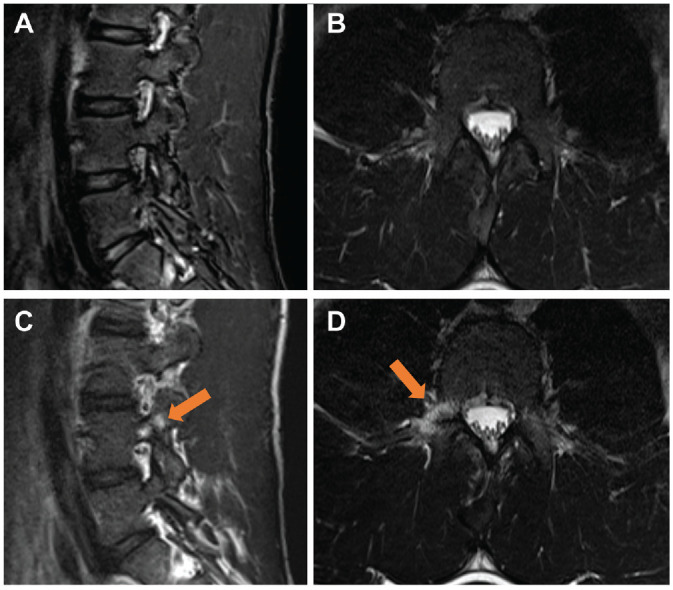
Sagittal Dixon and transverse T2-weighted magnetic resonance imaging scans of the lumbar spine of a 19-year-old competitive alpine skier (A and B, respectively) at baseline and (C and D, respectively) at the 48-month follow-up. Note the bone marrow edema in the right facet joint of the fourth lumbar vertebra (arrows), most likely due to overuse-related changes.
